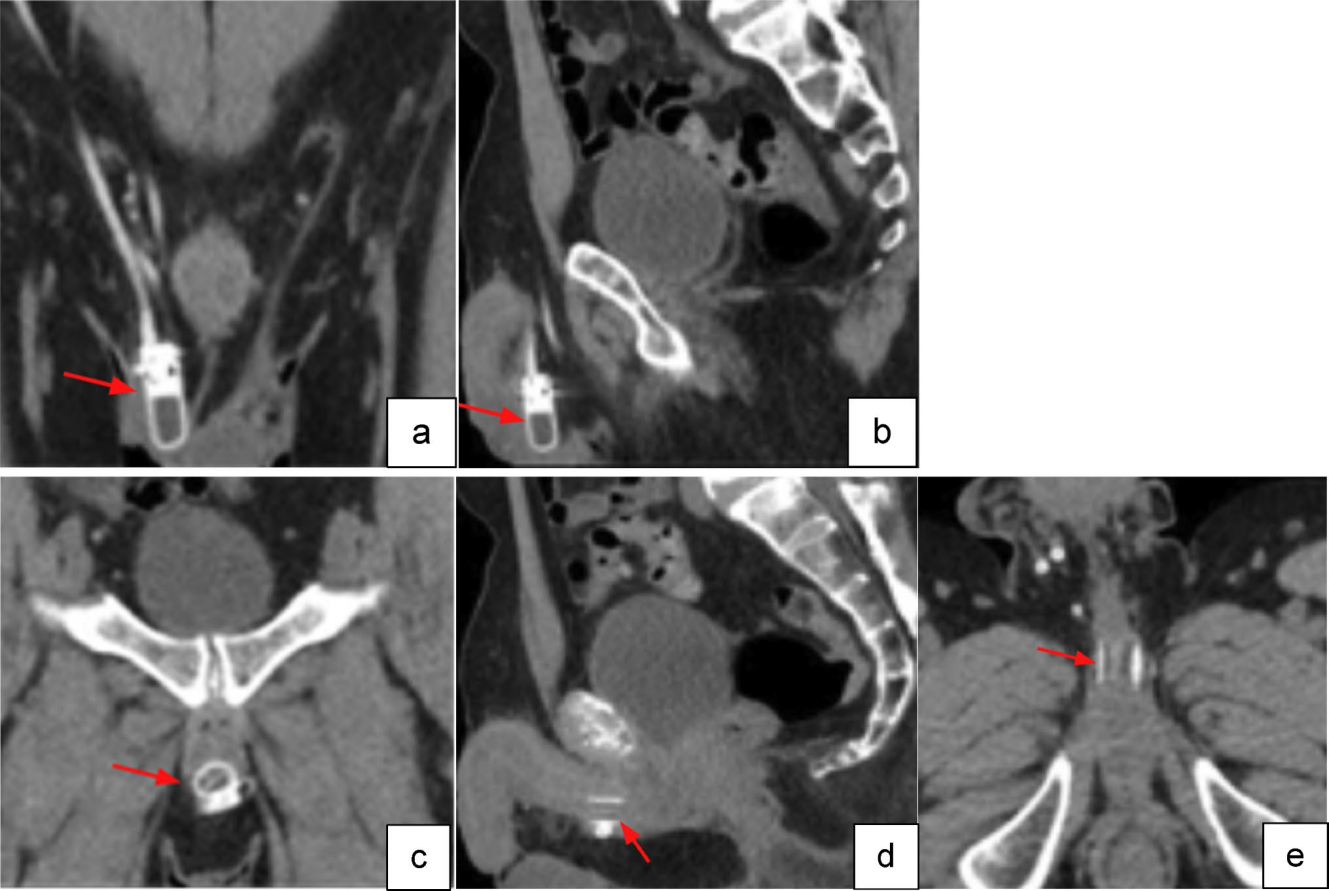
Coronal (a), and sagittal (b) non-contrast CT images of normal AUS pump location in the lateral scrotum (red arrow). Coronal (c), sagittal (d) and axial (e) non-contrast CT images of normal urethral cuff around the bulbar urethra (red arrows)
